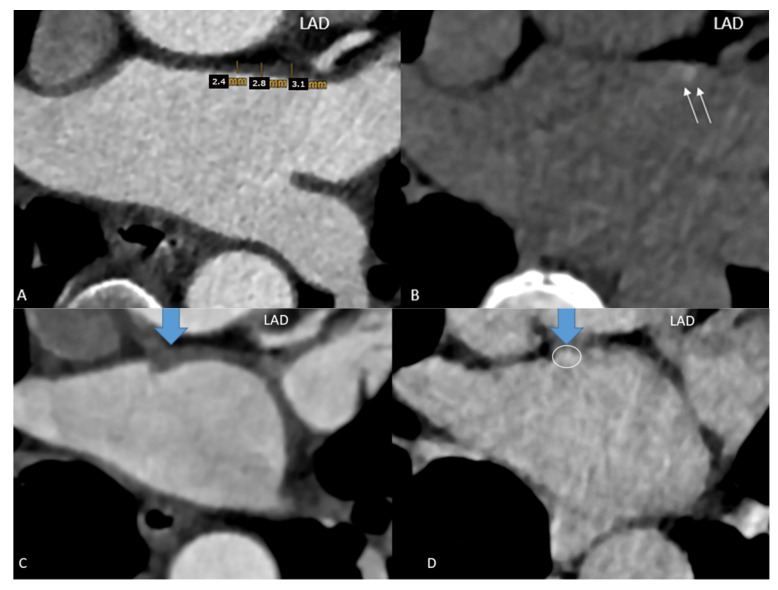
Focal late enhancement (LE). Images (A) of the early phase CTA were co-axially aligned with late enhancement CT performed 7 min after contrast agent injection (B) (2-step CT protocol). (A) LAWT was measured at 3 sites along the LA ridge, and the mean was calculated. The left anterior descending (LAD) coronary artery served as a landmark for co-axial alignment in this patient: a 52-year-old-female with persistent AF, a CVRF of active smoking. The LAWT was >2 mm (A) and focal LE with 130 HU (B) was observed. (C,D) Results in a 54-year-old male with AF prior to planning LA ablation. The mean LAWT was 4 mm (C), and a focal dense spot of late enhancement (LE) was identified at the exact site of maximal LAWT, with 102 HU (D).
